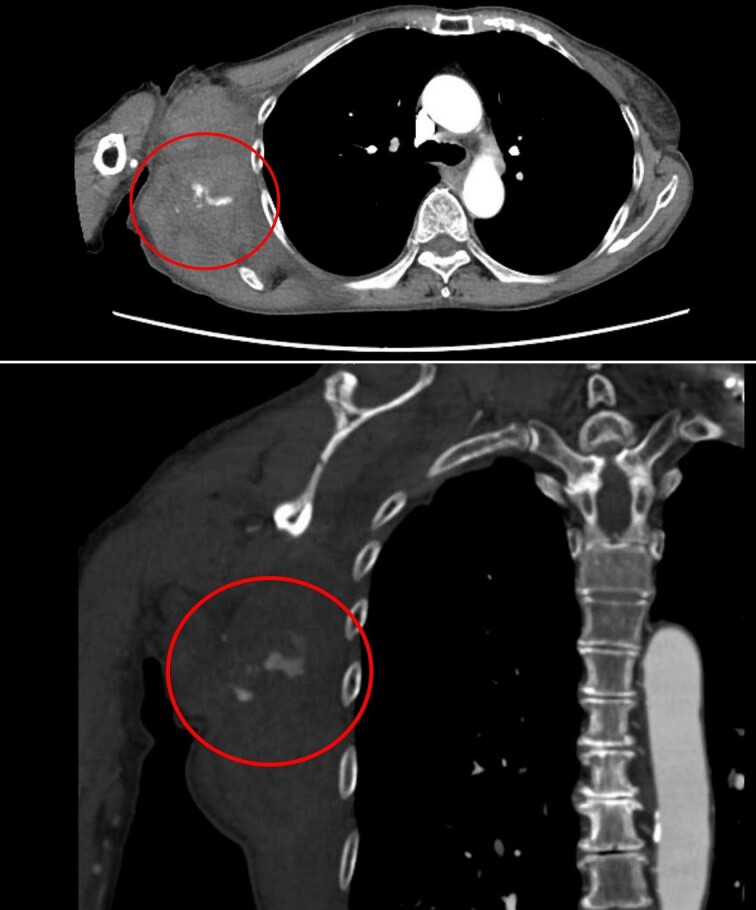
Contrast-enhanced CT at the time of the second bleeding showed growth of the hematoma and extravasation of contrast agent in a branch of the right lateral thoracic artery.
